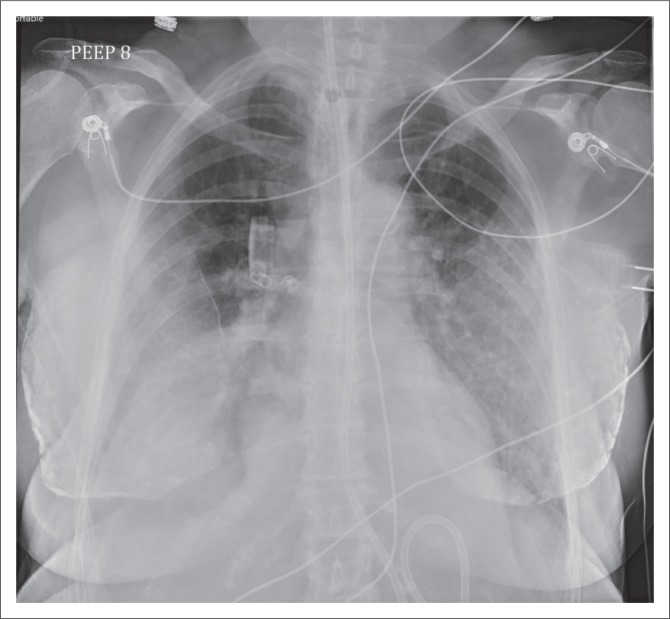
Chest radiograph showing heavily calcified breast implants obscuring the lung bases.
